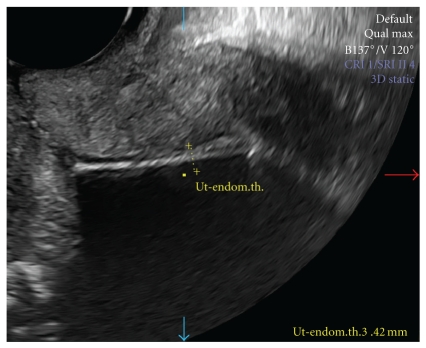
Sonographic view of uterus with LNG-IUS in situ. The endometrial thickness is 3.42 mm.
